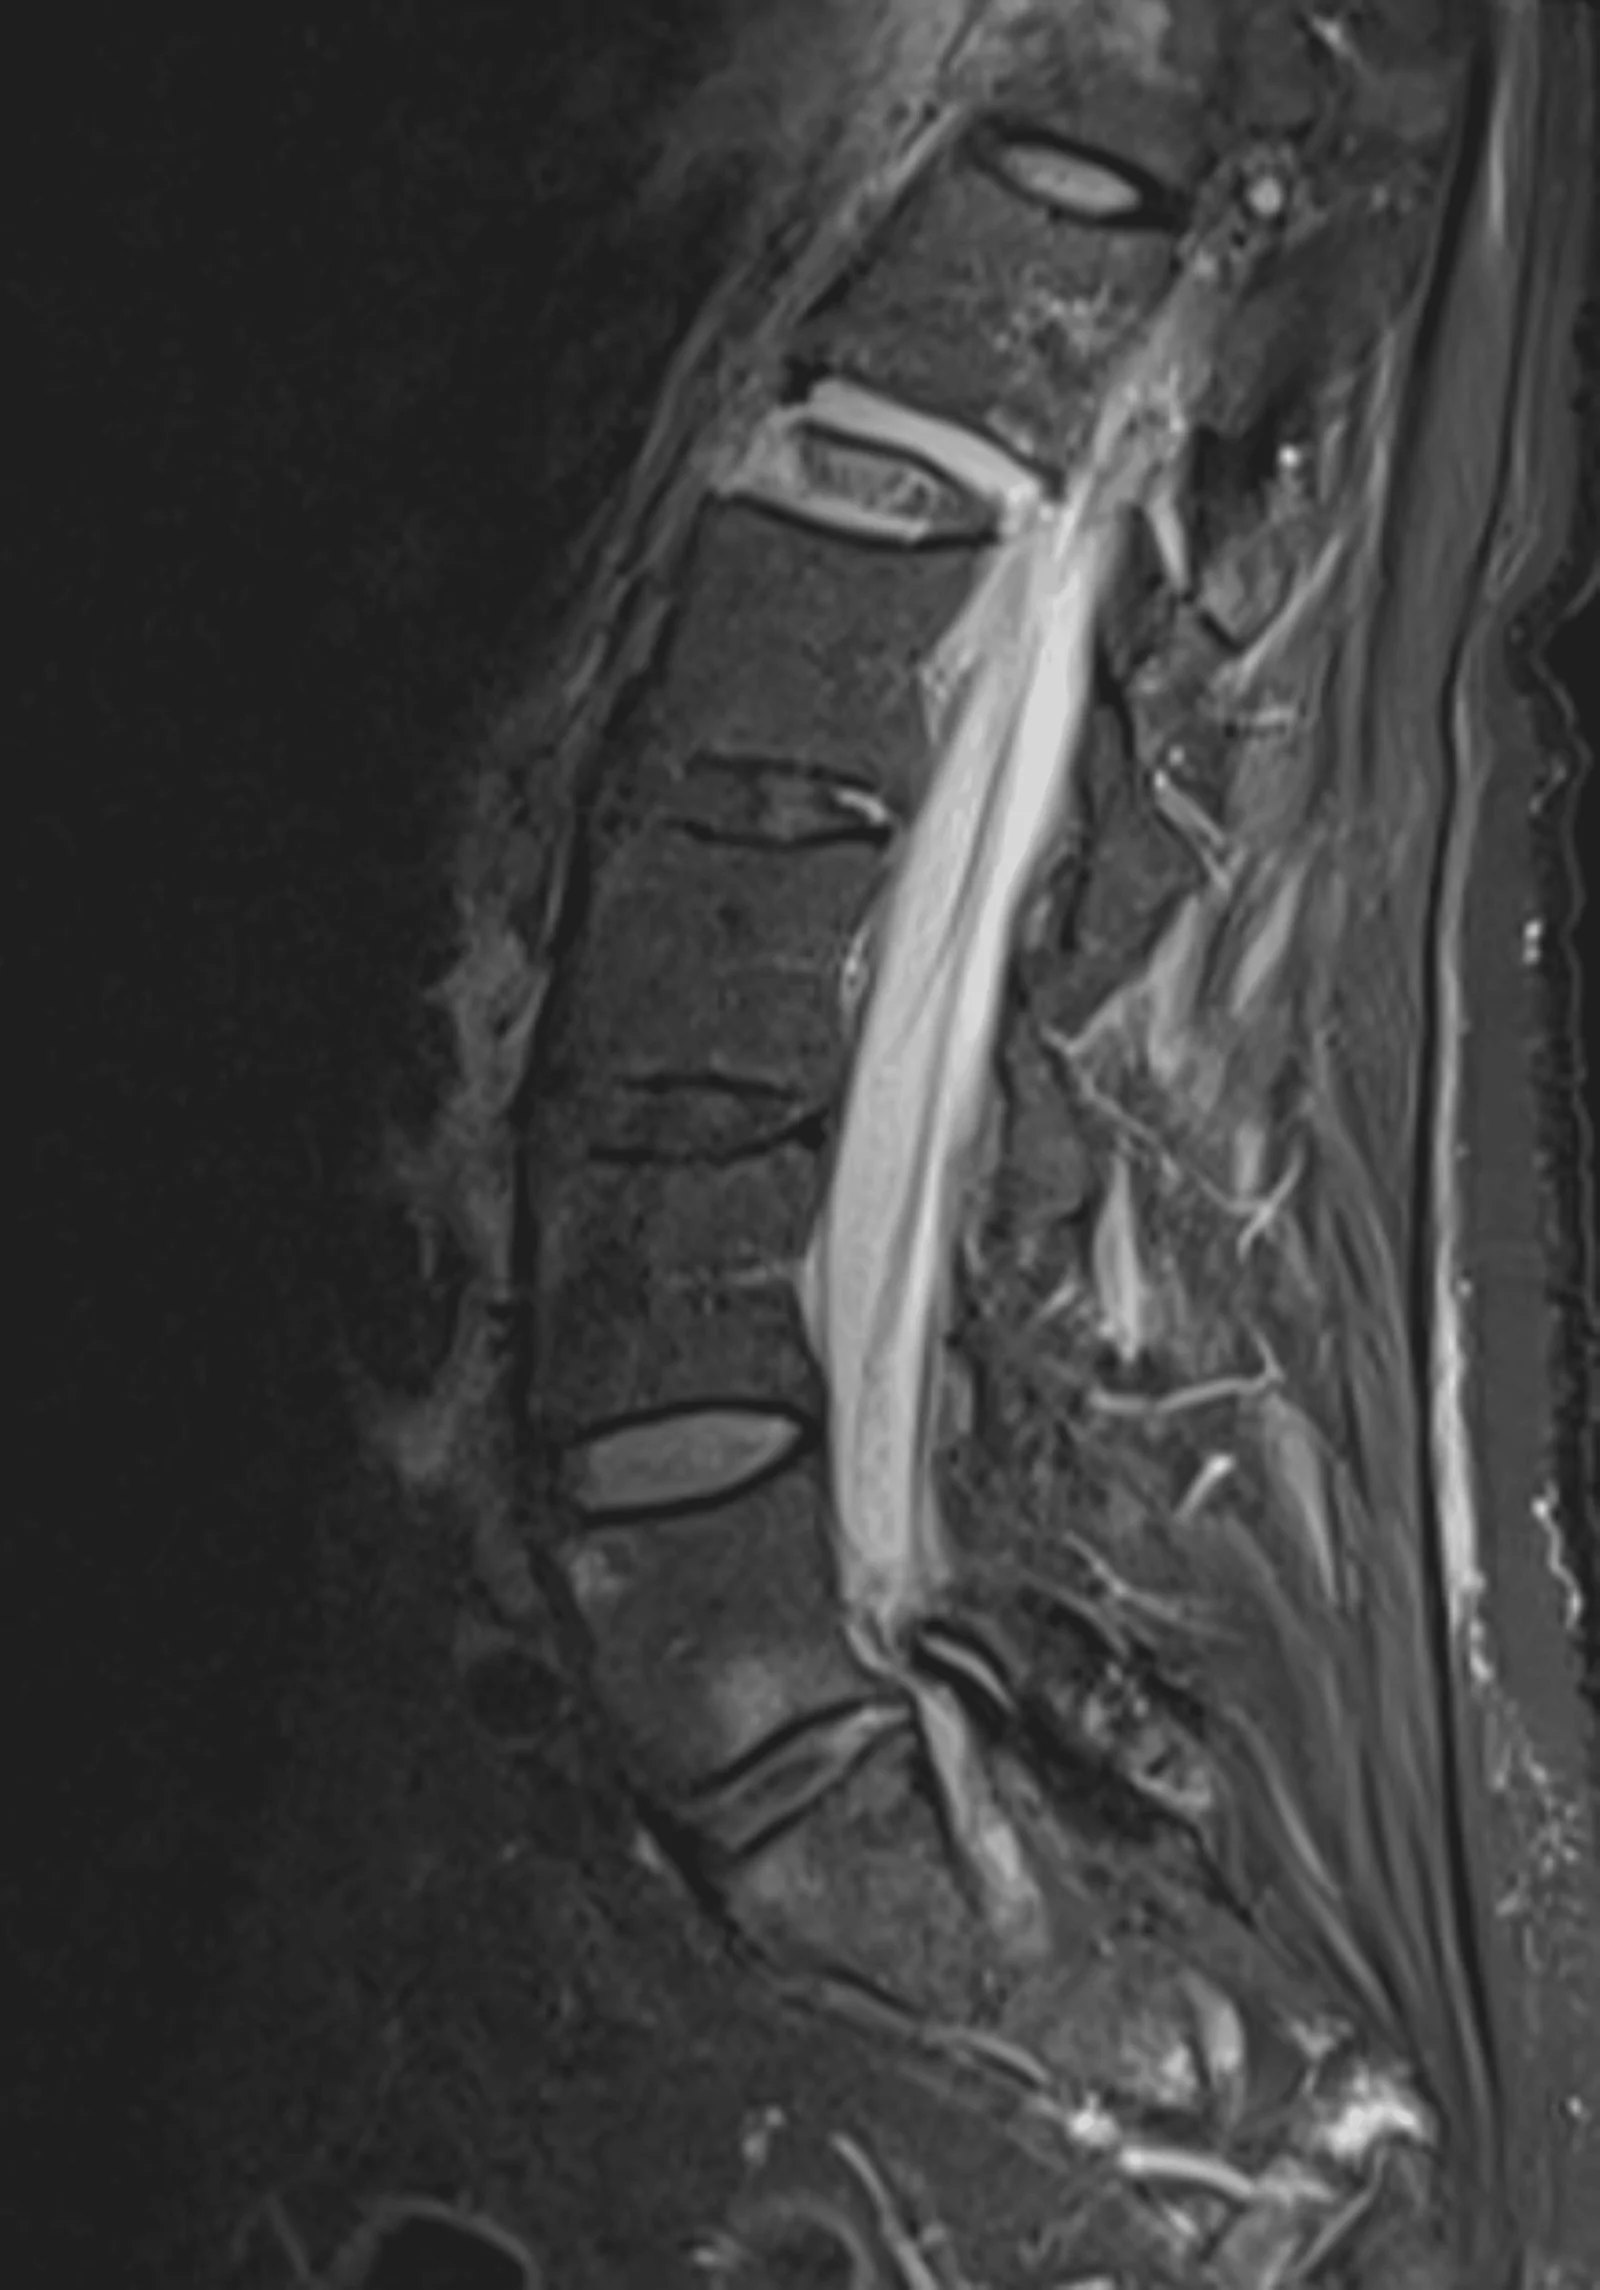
Sagittal STIR MRI demonstrates associated marrow edema and paraspinal soft-tissue injury. STIR, Short Tau Inversion Recovery.
